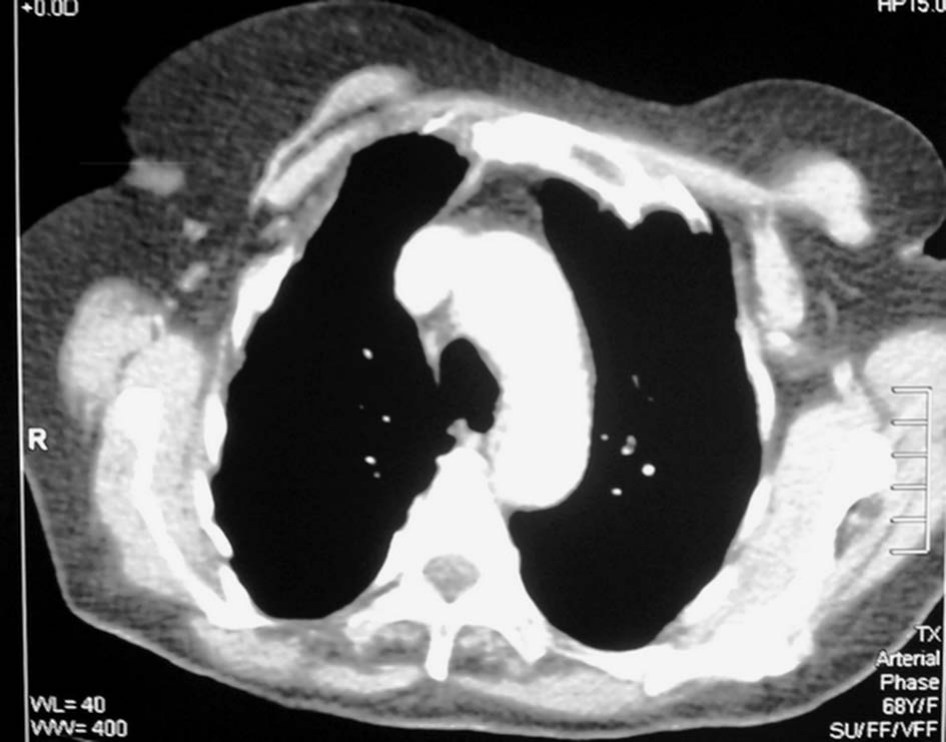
Postoperative Thoracic CT Image in Third Year
